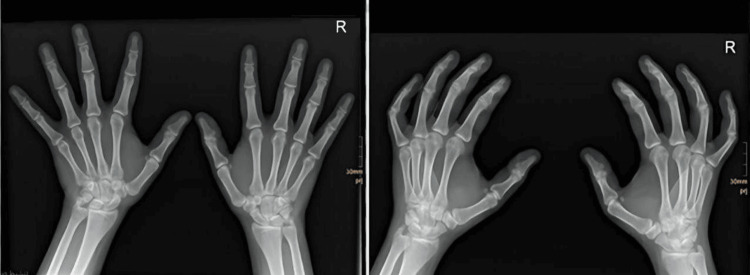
X-ray of the left hand showing early signs of healing.
